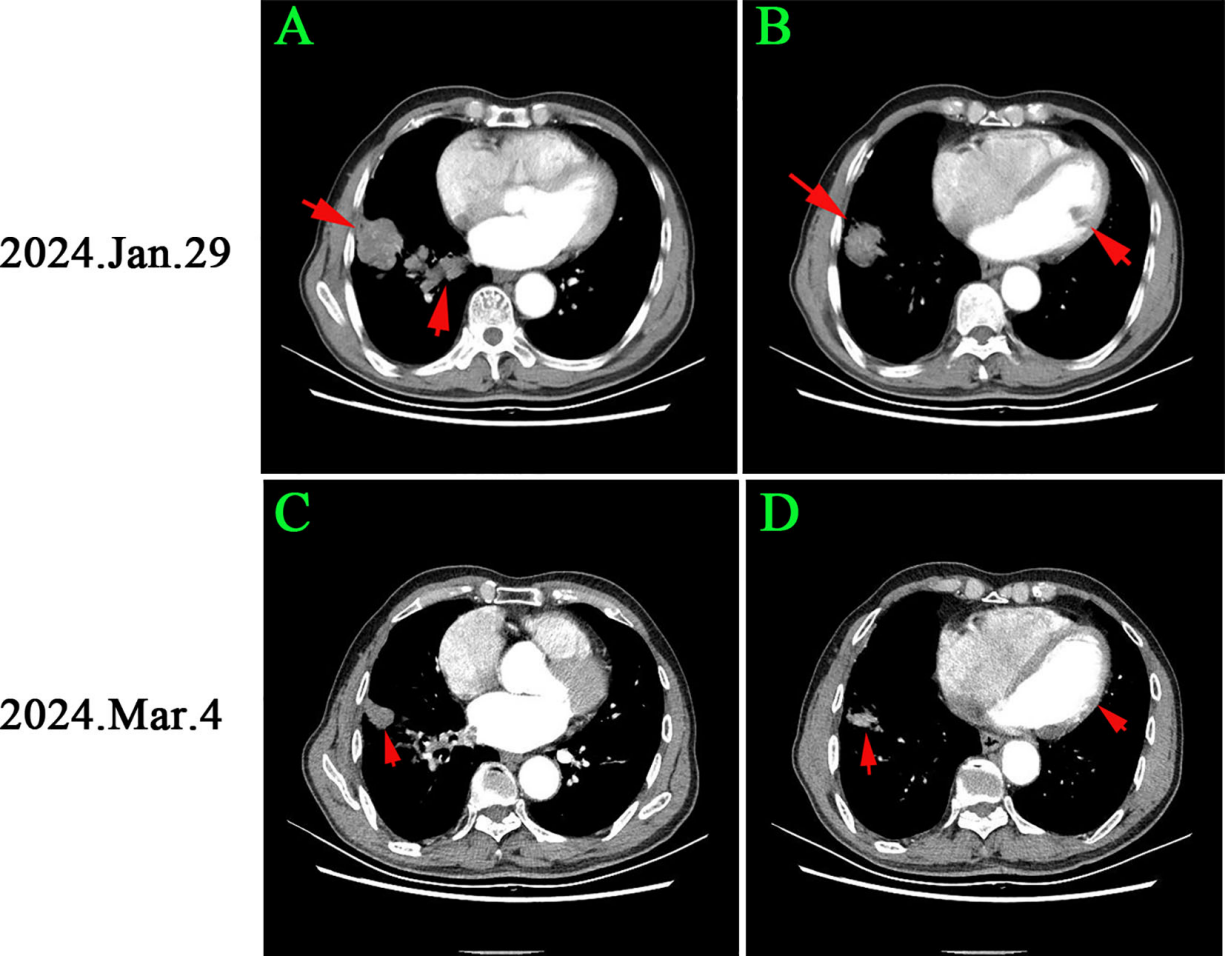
Chest CT changes before and after osimertinib treatment: (A, B) Initial imaging showed a 2.8 cm right lower lung mass and multiple pulmonary nodules, with a clot in the right inferior pulmonary vein. (C, D) After 1 month of osimertinib, the lung cancer lesion reduced to 1.2 cm, and the pulmonary nodules significantly decreased, with no filling defects in the vein. According to RECIST criteria, the patient achieved part remission (PR).
